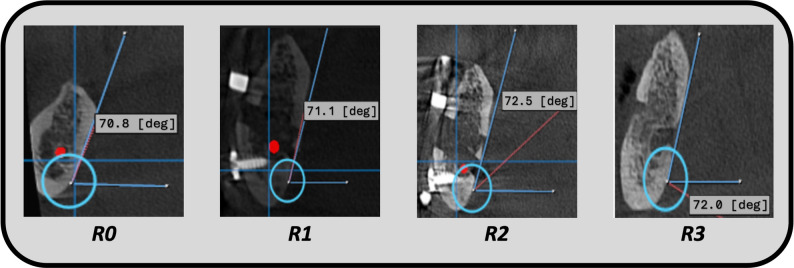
Lingual displacement tilt angle assessment across the radiographic timeline of the study. The tilt angle is calculated between the basal bone and movable segment from the lingual side in the cross-section view of the CBCT record
